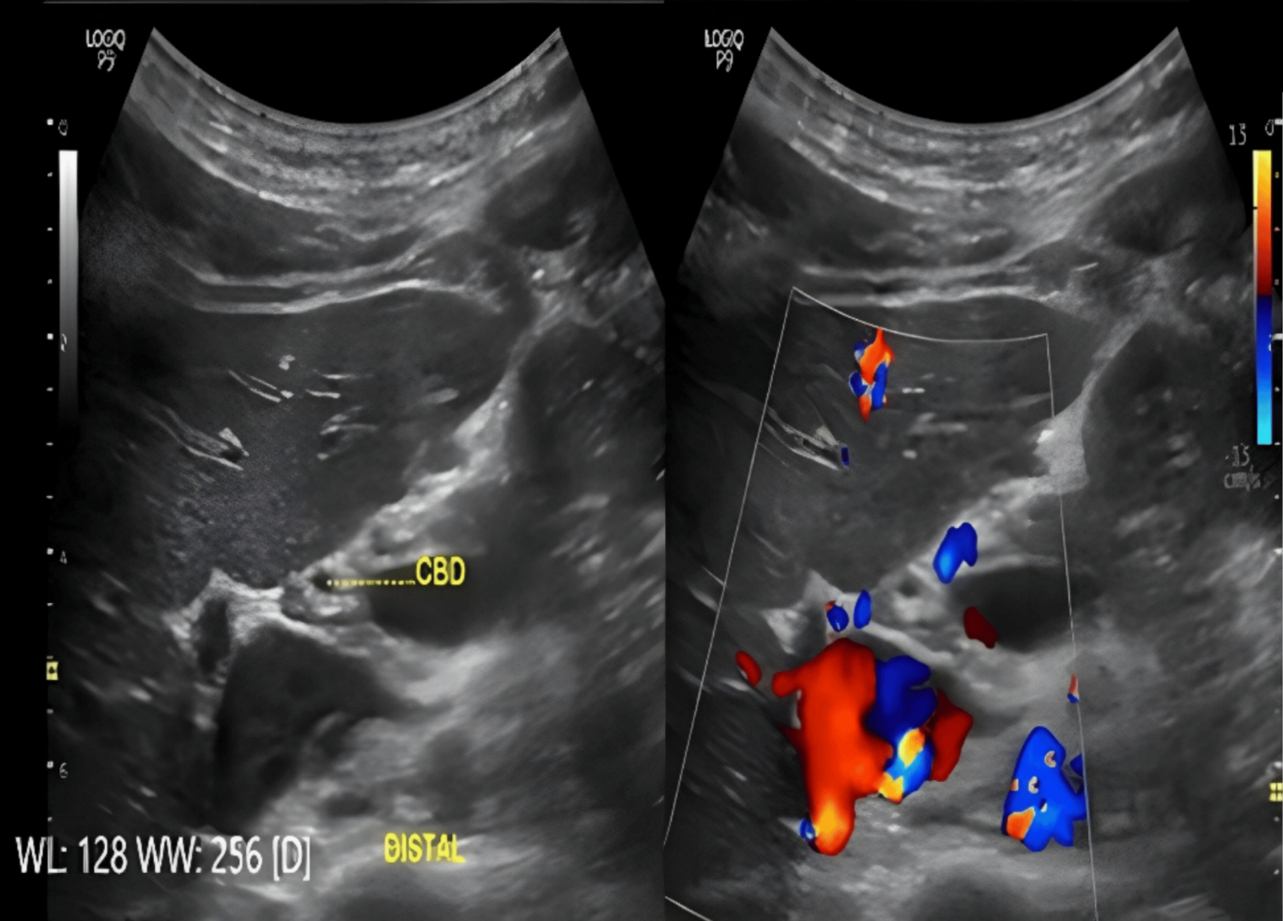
Ultrasonographic measurement of the distal portion of the common bile duct (CBD) Representative cross sectional sonographic image obtained at the distal portion of the CBD in the head of the pancreas. This figure highlights the third anatomical level used in the study’s protocol.
